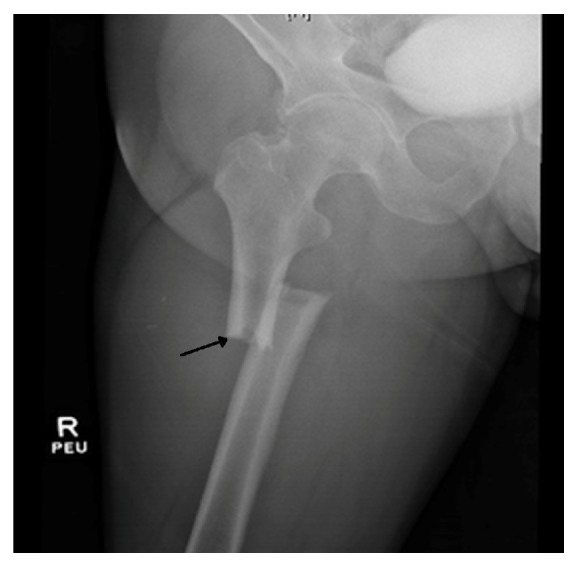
Right Femur X-Ray showing acute femur fracture (black arrow).
